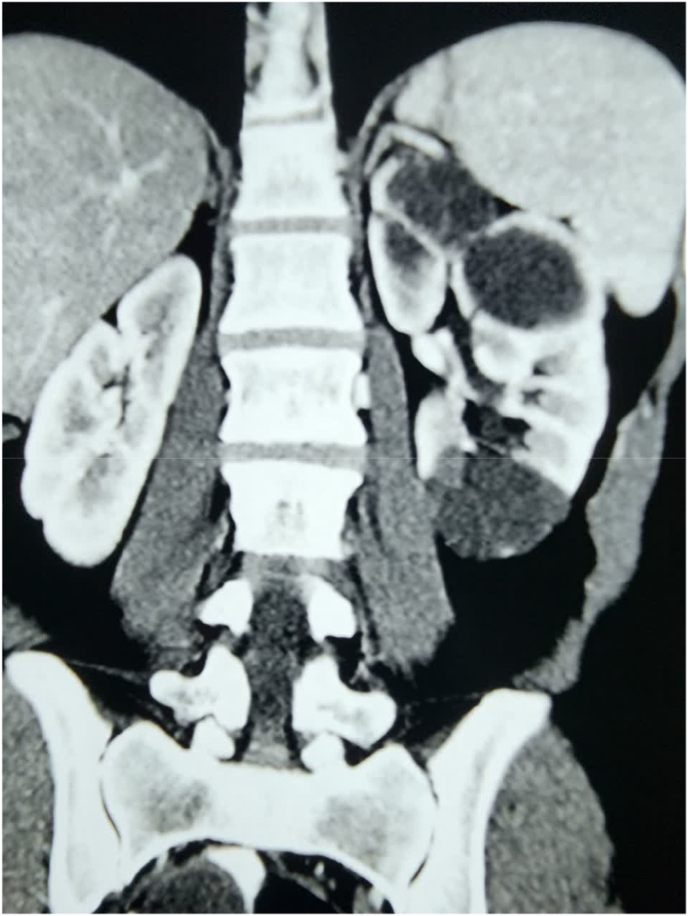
Preoperative CT scan revealed two separate well-circumscribe masses on the upper pole and lower pole of the left kidney with mixed solid and cystic components that contain thick septa with delayed contrast enhancement.
